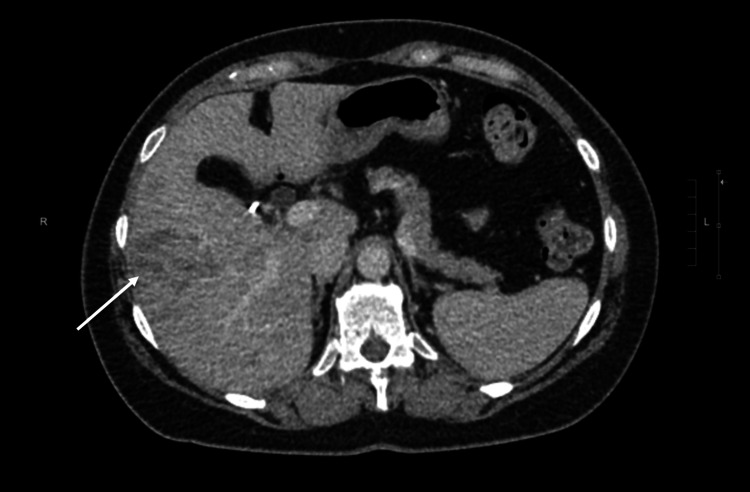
CT abdomen from case two showing metastasis in the right lobe of the liver.
